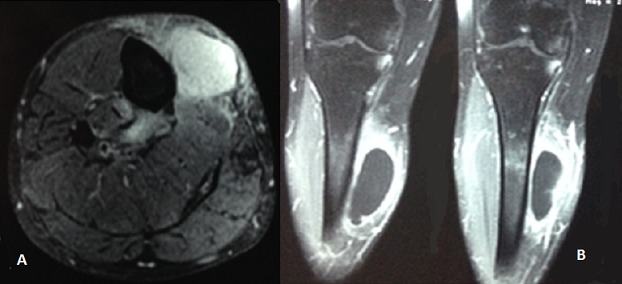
MRI of the right leg, (A) axial T2-weighted: subcutaneous and tibialis posterior cysts; (B) coronal T1 FAT SAT GADO: subcutaneous cystic structure.
